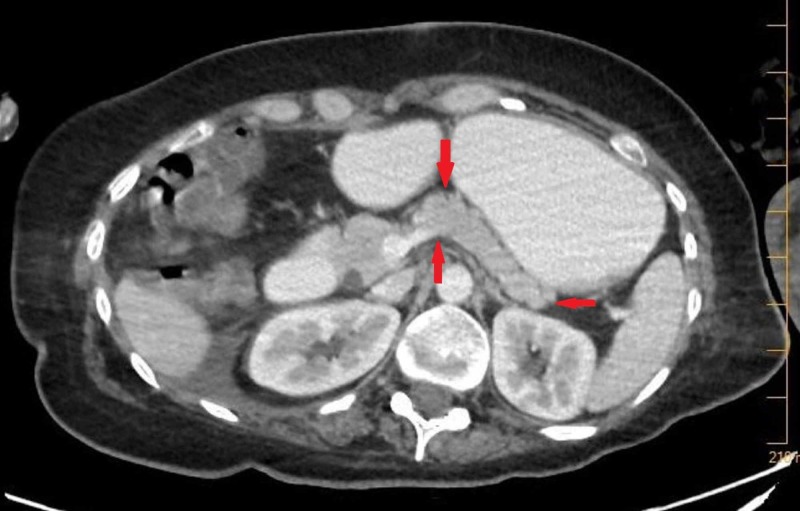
CT scan of the abdomen with contrast showing the normal pancreas without any pancreatitis or pancreatic lesions.
